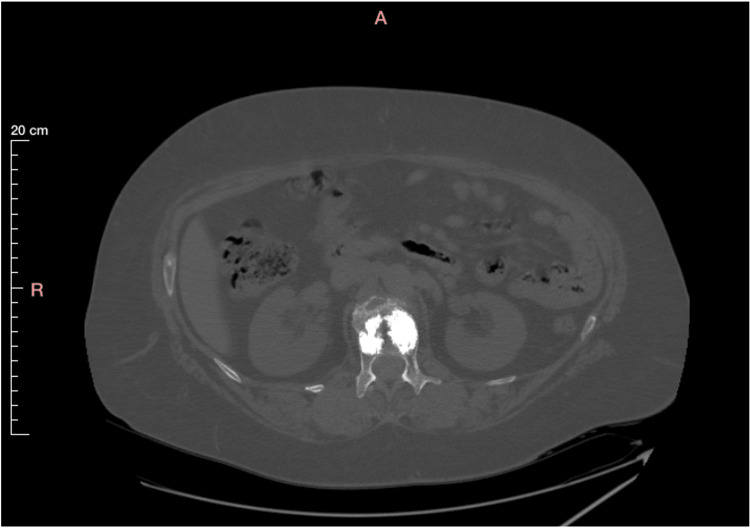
CT scan (axial view) of the lumbar spine obtained at four months following the two balloons kyphoplasty. No gross signs of a malignant tumor at the L1 vertebra, and/or breaching of the vertebral cortex are apparent.
